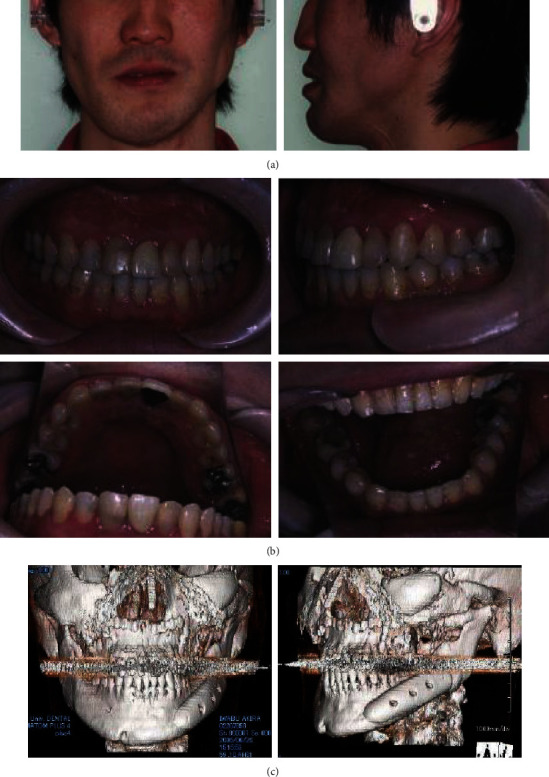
Facial (a) and intraoral photographs (b) and CT images after postoperative orthodontic treatment (c).
